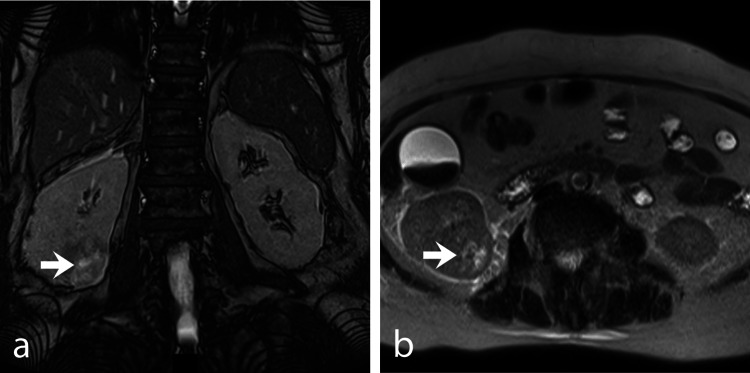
Contrast-enhanced abdominal magnetic resonance imaging. a) Mass in the lower pole of the right kidney, coronal section. b) Axial section showing the same mass in the lower pole of the right kidney.
